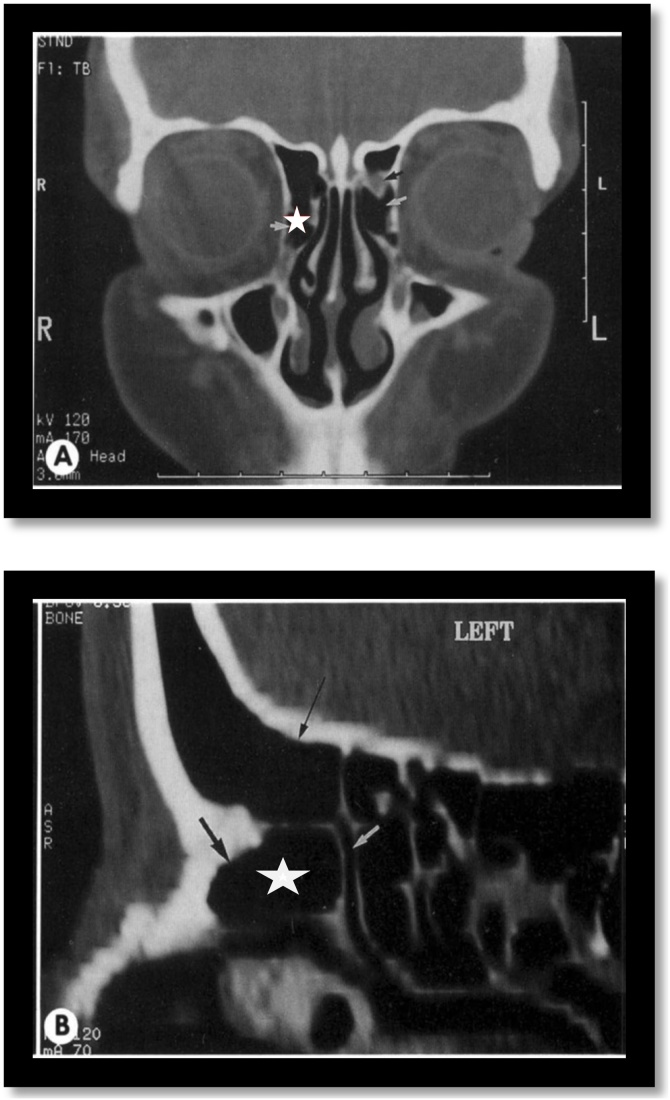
Agger nasi cell. Coronal and Sagittal computed tomography sections demonstrate Agger nasi cell (white star).
